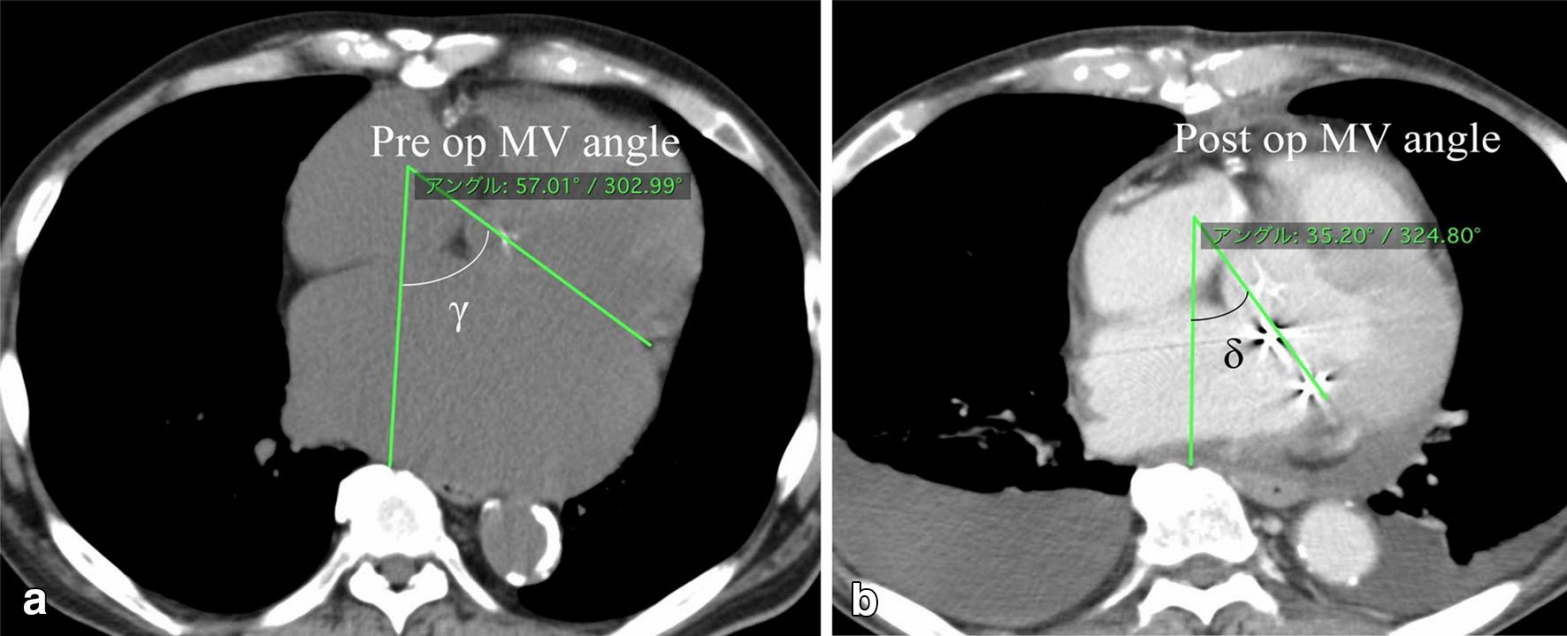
Pre and post-operative computed tomography. Mitral valve (MV) angle indicate the angle between mid-sagittal plane and the mitral annular plane. a The γ indicates the preoperative MV angle. b The δ indicates the post-operative MV angle
